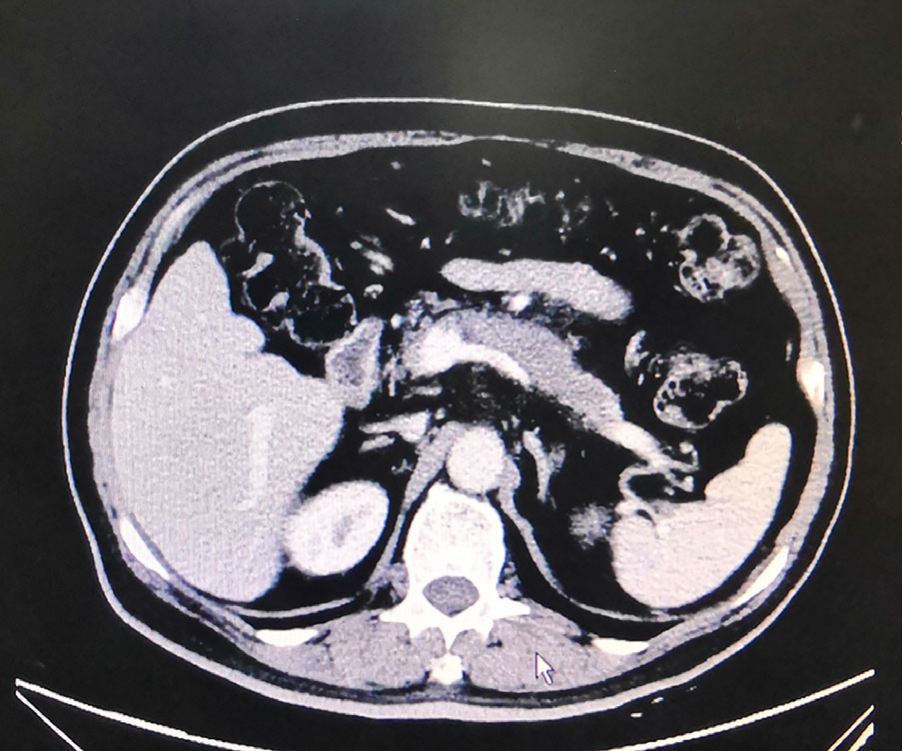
Upper abdomen routine enhanced CT scan of the diabetic patient.
